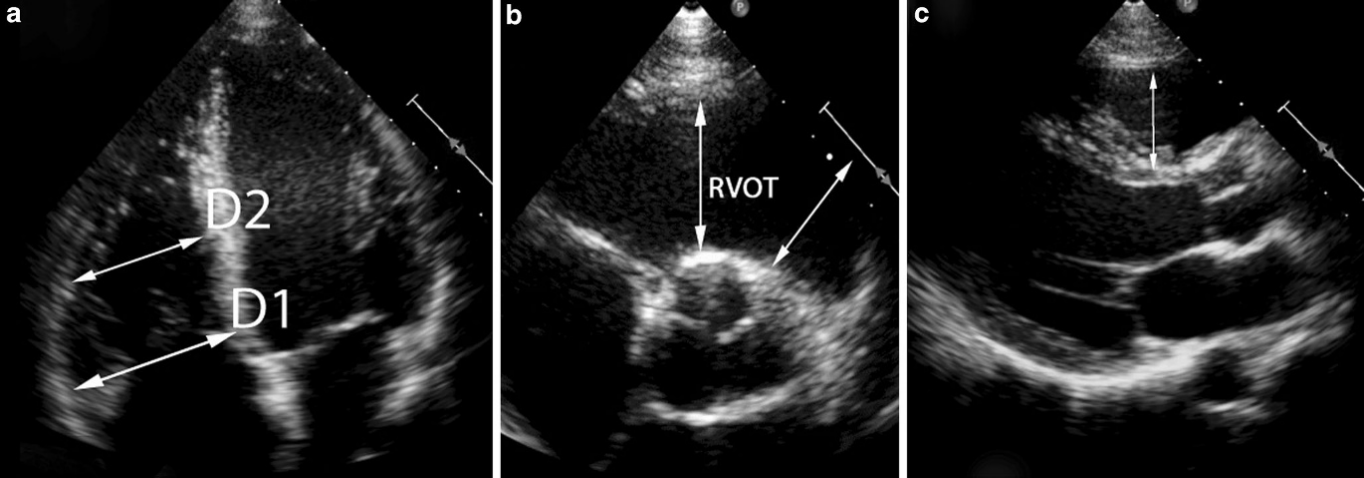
Measurements of RV dimensions. The RV basal (D1) and mid cavity (D2) dimensions are shown in the RV focused apical four-chamber view (a). Measurements of the right ventricular outflow tract at the proximal level and the distal level are shown in the parasternal short-axis (b) and parasternal long-axis view (c)
